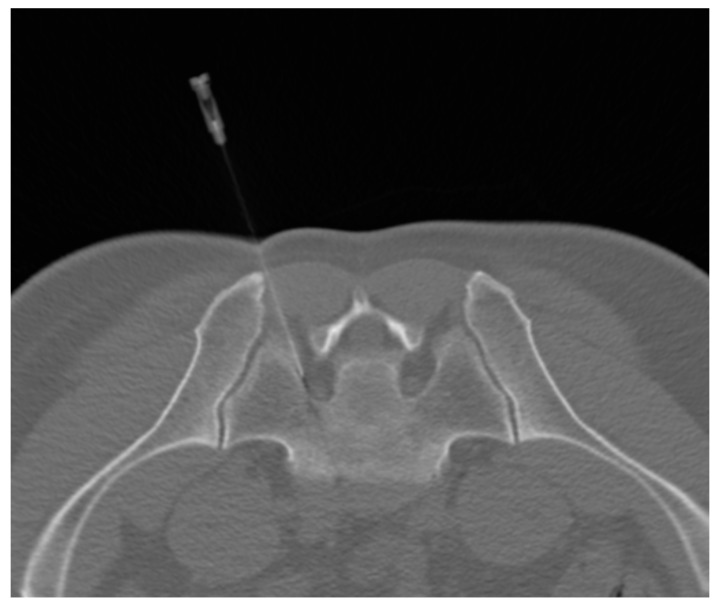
A transverse low-dose CT scan demonstrates the positioning of the radiofrequency cannula adjacent to the dorsal root ganglion of the left-sided S1 nerve root.
